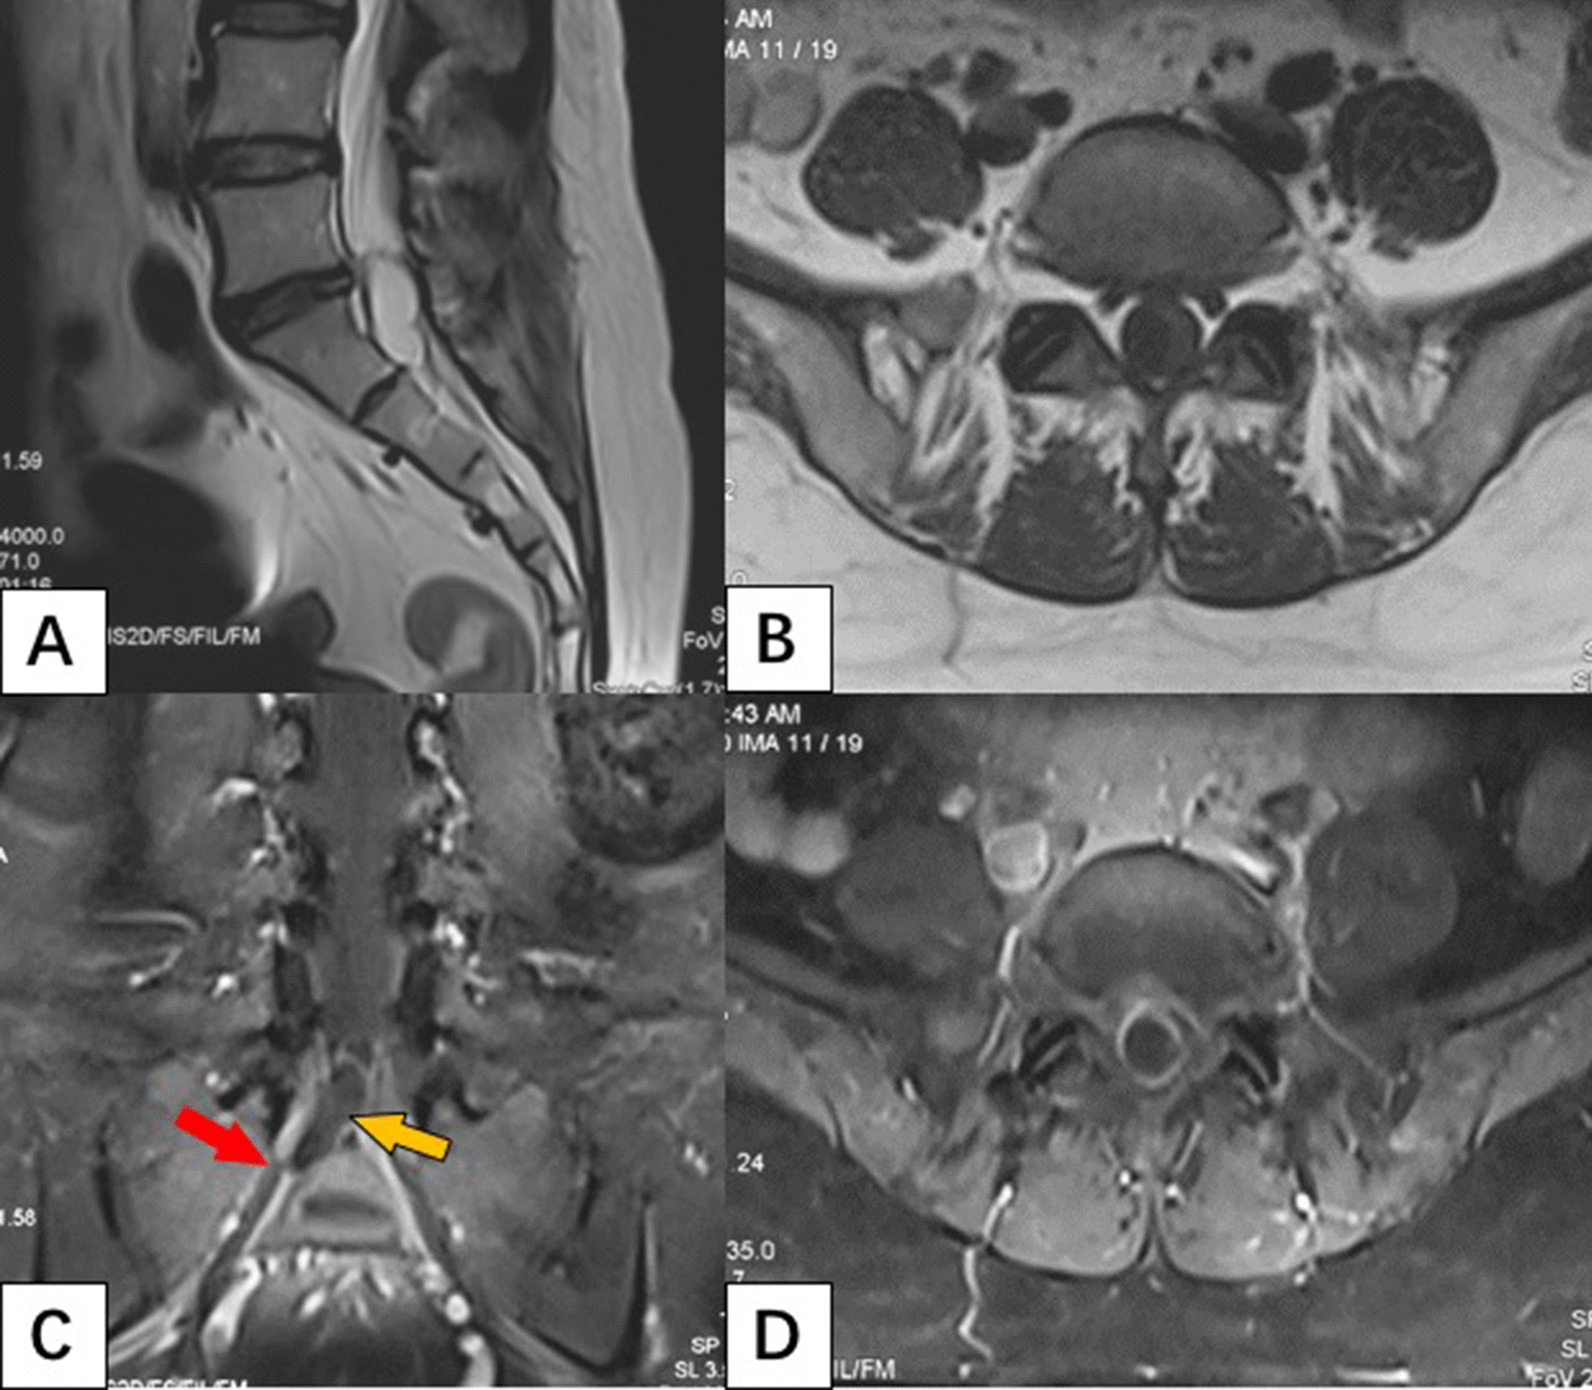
Sagittal T2-weighted MR image showing a relatively well-defined cystic intramedullary lesion on lumbosacral spine with hyperintense on T2WI (A). The lesion was located to the right of the spinal canal on axial T1-weighted MR with hyperintense T1WI (B). Coronal-enhanced T1-weighted MRI scan showed that most of the lesions (yellow arrow) were located under the dura, and some penetrated with the sleeve of L5 nerve (red arrow) (C). Axial-enhanced T1-weighted MR showed thin enhancing peripheral wall (D)
